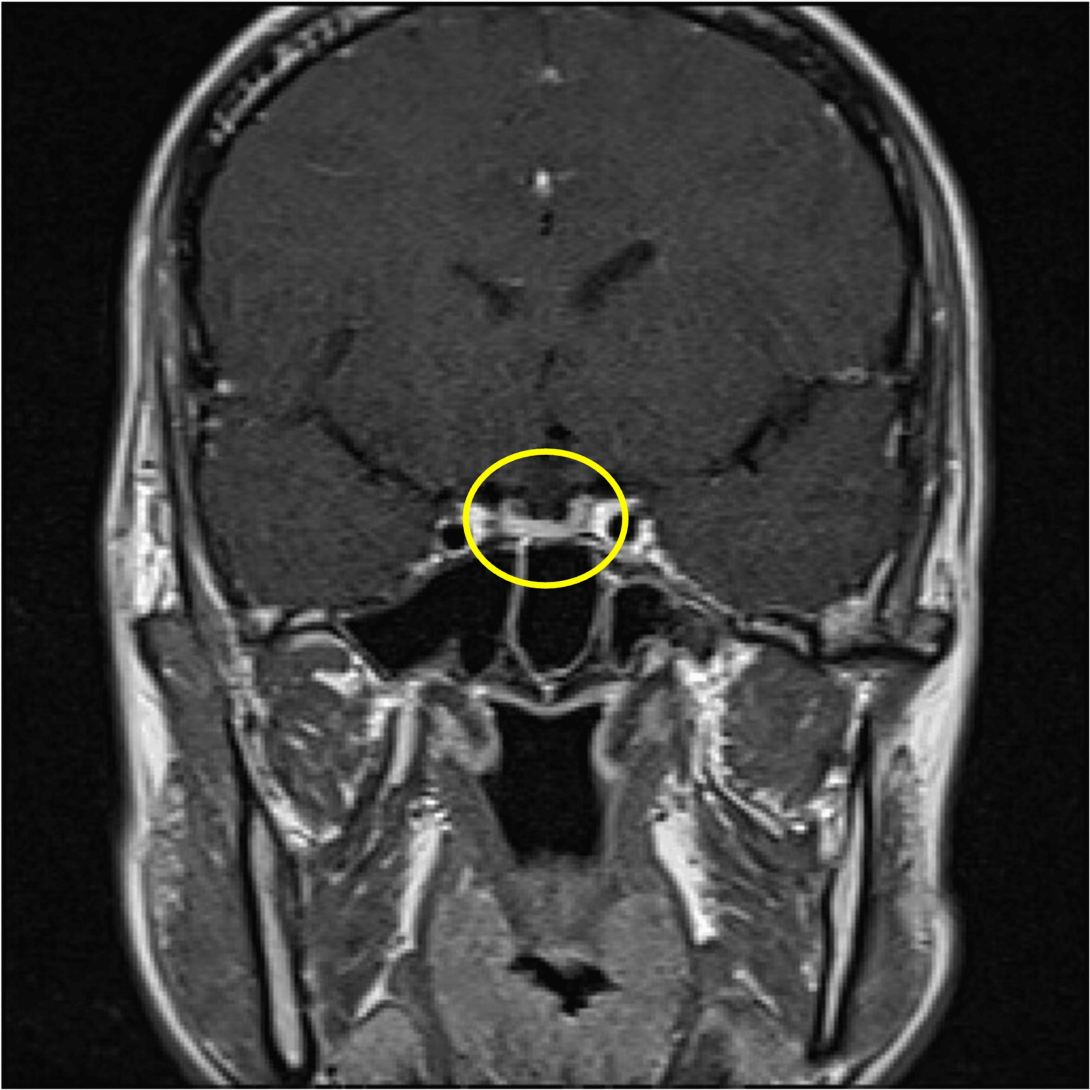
Contrast-enhanced MRI scan of the brain Coronal contrast-enhanced MRI (late phase). The pituitary region reveals no abnormal enhancement or structural distortion, consistent with absence of macroadenoma.
